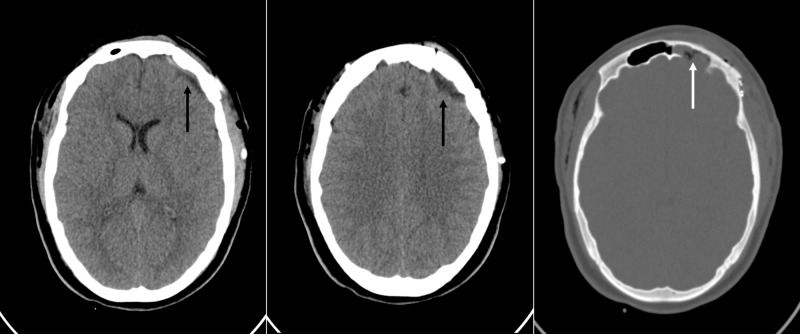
Postoperative CT scans of the head Postoperative contrast-enhanced computed tomography (CT) of the head. Note in the left and middle images the absence of a residual lesion (black arrow) on the axial slices with the brain window and, in the right image, with the bone window, the post-operative cranialization of the left frontal sinus with the filling of the cavity with a periosteum graft (white arrow).
